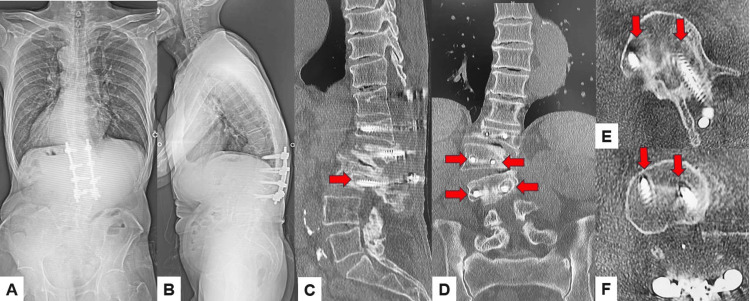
Postoperative images A: Posteroanterior whole spinel radiogram, B: Lateral whole spine radiogram, C: Midsagittal lumbar reconstruction CT, D: Coronal lumbar reconstruction CT, E: Axial CT at L2, and F: Axial CT at L3. Red arrows indicate screw loosening.
